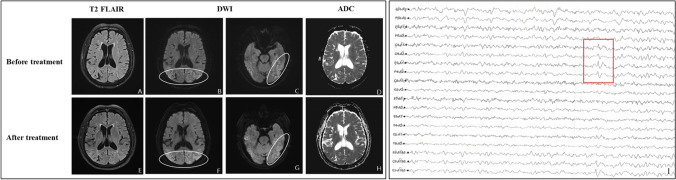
Brain MRI and EEG in Patient 8. A–D show brain imaging examined before treatment, and E–H show imaging during the follow-up period. B, C, F, G Hyperintense signal presented along the bilateral parietal occipital lobe cortex on DWI; D, H hypointensity presented in the same lesions as B, F on ADC imaging. A, E The lesions presented slightly hyperintense signal. E, F The lesions after treatment were more extensive than those before treatment (A, B). I EEG showed an increase in slow waves diffusely, and suspicious three-phase waves (red rectangle) were observed in the bilateral parietal, central and right occipital regions
